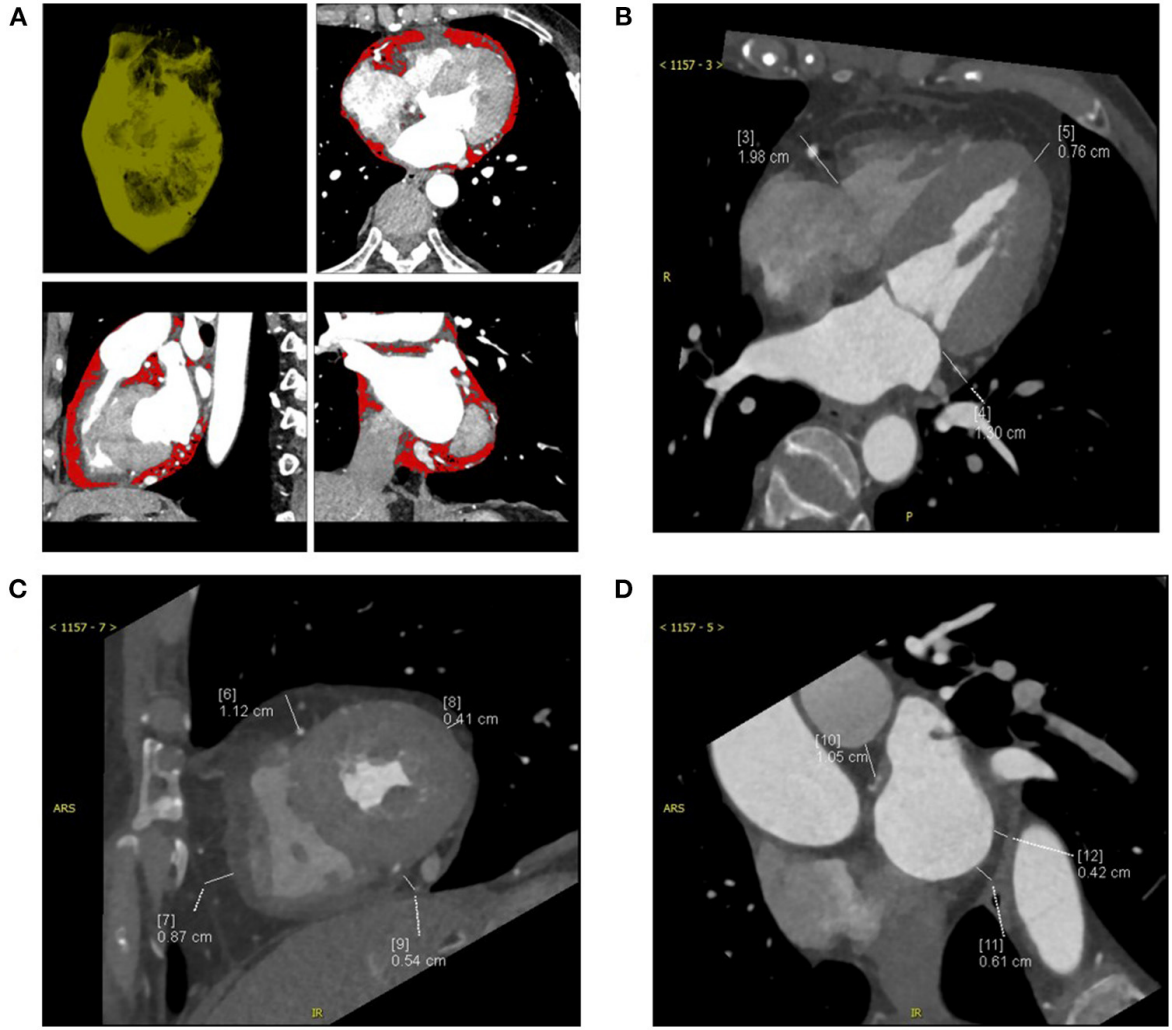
The computed tomography (CT) measurements of a patient with CRT-responsive participating in this study were shown and epicardial adipose tissue (EAT) was measured by multidetector CT. (A) Contrast-enhanced CT scan presenting the region of interest (ROI) and pixels attributed to epicardial adipose tissue (red areas). (B) EAT thickness was measured on the parasternal short-axis view. (C) EAT thickness was measured on the horizontal long-axis view. (D) EAT area was measured at the left main coronary artery level. CRT, cardiac resynchronization therapy.
